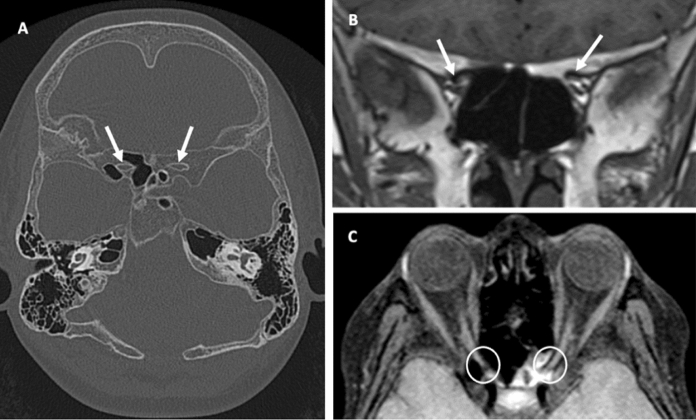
Selected neuroimaging views from the patient. Axial head computed tomography (CT) (A) demonstrates generalised expansion of the diploic spaces causing secondary narrowing of the optic canals which is fairly symmetrical (arrows). Coronal T1 weighted magnetic resonance imaging (MRI) (B) and axial fat-suppressed T1 weighted MRI (C) show flattening and attenuation of the intracanalicular aspects of the optic nerves bilaterally (arrows)
